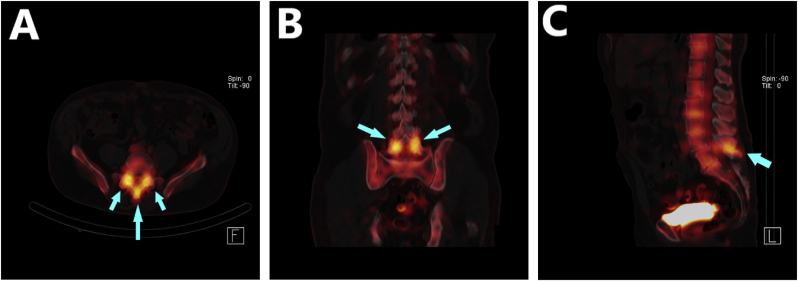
NM Bone Three-Phase Study with SPECT. Axial (A) and coronal (B) views reveal intensive radiotracer uptake within the bilateral L4-L5 facet joints. Sagittal view (C) indicates pronounced radiotracer activity in the L4-L5 interspinous bursa. Basstrup disease, commonly referred to as “kissing spine,” is evident at this anatomic level. Light blue arrows indicate areas of increased radiotracer uptake. (For interpretation of the references to colour in this figure legend, the reader is referred to the Web version of this article.)
